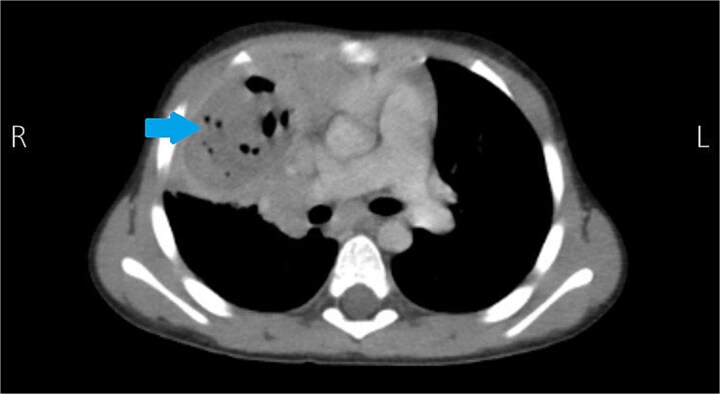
Contrast-enhanced CT showing right upper lobe cavitary lesion consistent with ruptured hydatid cyst.
